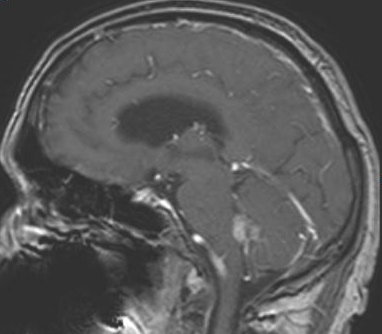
Sagittal contrast enhanced magnetic resonance image of 4th ventricle choroid plexus carcinoma
